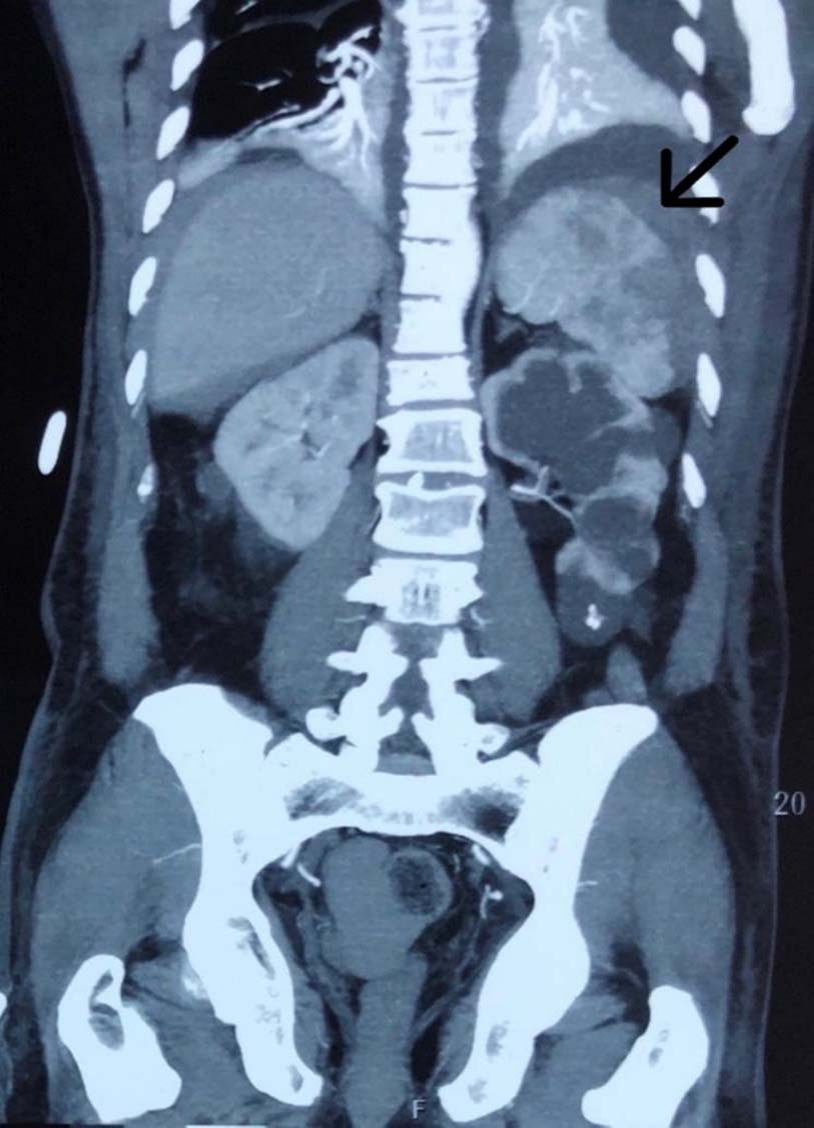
Contrast enhanced computed tomography scan of abdomen (coronal view) showing grade II splenic hematoma.
